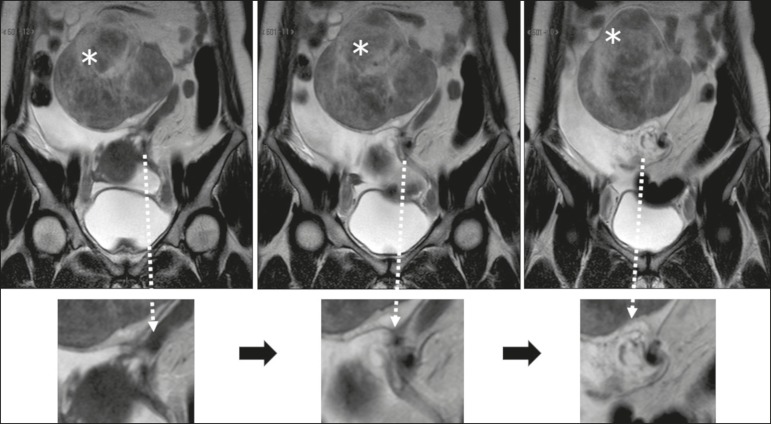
Coronal T2-weighted MRI sequences, with magnified images below. Note that the ovarian pedicle is twisted around its own axis. The left ovary is enlarged due to the presence of a hypointense, heterogeneous expansile formation consistent with a fibroma (asterisks).
